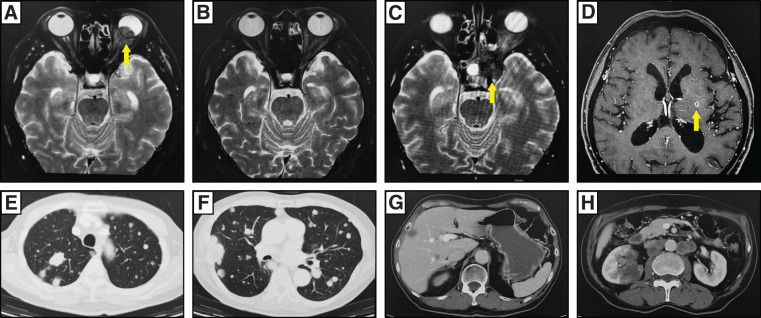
Images during treatment. The treatment progress of metastatic lesions in the head was compared using MRI. At 19 months after ocular metastasis, the metastatic lesion increased in size (A, yellow arrow). At 22 months, the metastatic lesion had almost disappeared (B). At 28 months, the metastasis had infiltrated the orbit, skull, and paranasal sinuses (C, yellow arrow). At 29 months, metastasis was detected in the left basal ganglia (D, yellow arrow). Multiple systemic metastases were identified by CT scan. Multiple metastatic lung tumors were found throughout both the lungs (E and F), with liver metastasis (G), right kidney metastasis, and para-aortic lymph node metastasis also present (H).
